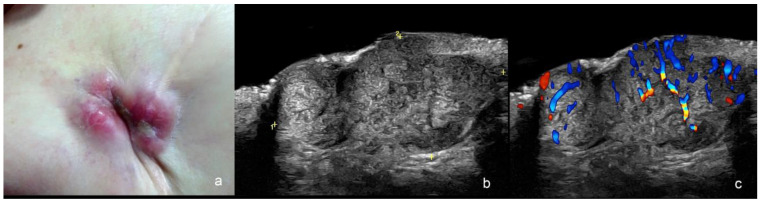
(a–c). Cutaneous metastasis of breast cancer in the scar of the mastectomy. (a). Clinical image. (b,c). Ultrasound images at 24 MHz ((b), greyscale and (c), color Doppler) present a hypoechoic dermal and hypodermal mass (between markers in (b)) with lobulated borders and hypervascularity on color Doppler (c).
